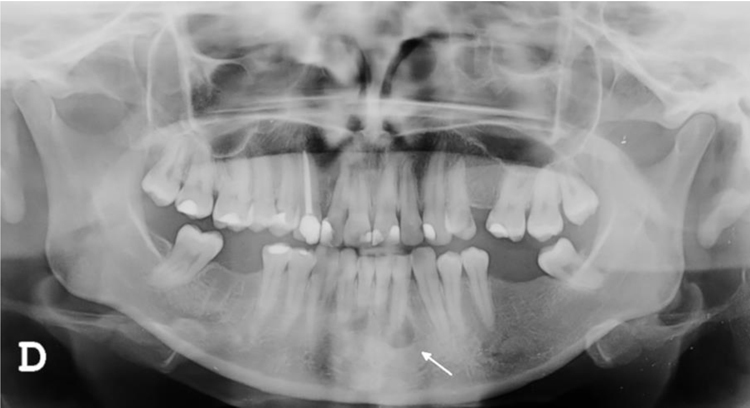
Panoramic radiograph showing radiolucency around the root of the mandibular left lateral incisor (arrow).
